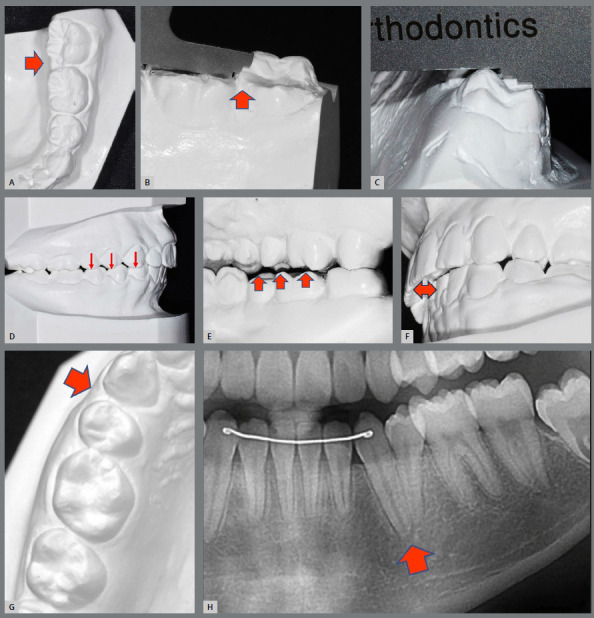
Cast-Radiograph Evaluation (CRE) criteria, which detected errors in the following categories: A) alignment, B) marginal ridge leveling, C) buccolingual inclination, D) occlusal relationship, E) occlusal contacts, F) overjet, G) interproximal contacts, and H) root parallelism.
